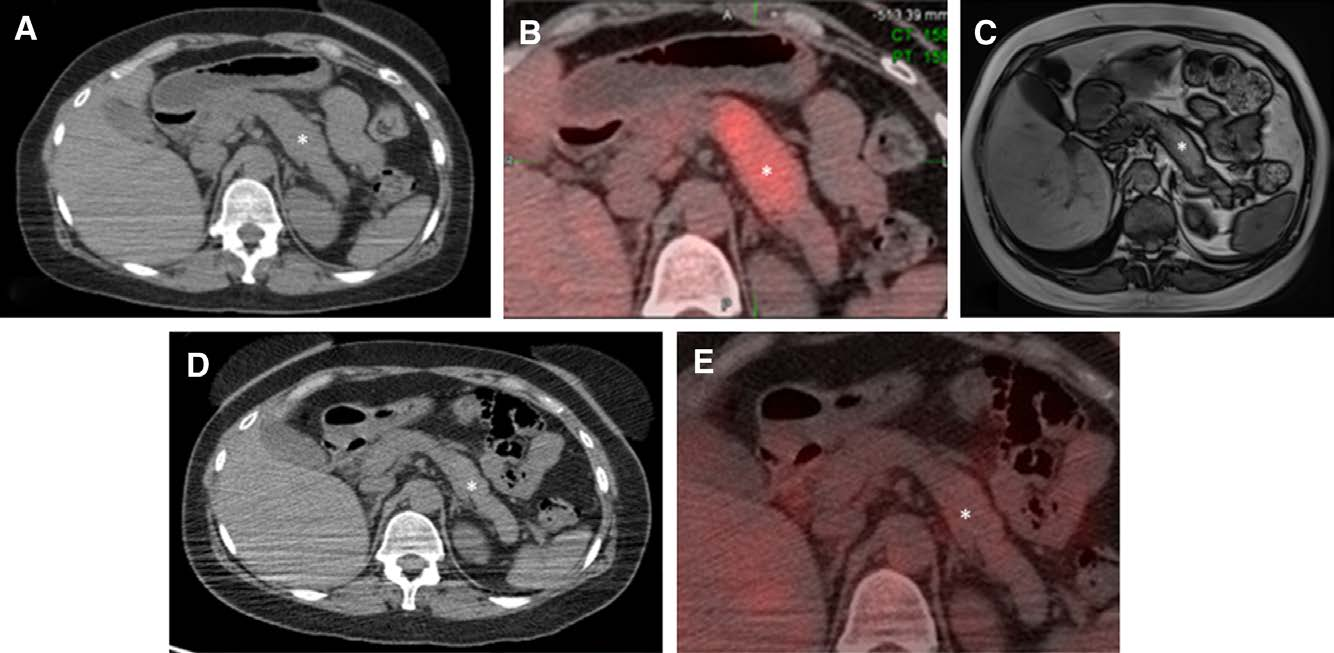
Images of pancreatitis. (A) PET/CT scan from October 2020 showing pancreatic swelling consistent with pancreatitis. (B) PET scan from October 2020 showing standardized uptake values of 6.6 to 8.1 in the pancreas. (C) T1-weighted magnetic resonance image of the abdomen acquired in the axial plane in December 2020. The pancreas overall appears smaller in diameter than that on PET/CT performed in October 2020 (A), consistent with resolving pancreatitis. (D), PET/CT scan from June 2021 showing near resolution of diffuse pancreatic swelling. (E) PET scan from June 2021 showing decreased pancreatic standardized uptake values (2.3–3.3). Asterisks indicate pancreatitis. CT = computed tomography, PET = positron emission tomography.
